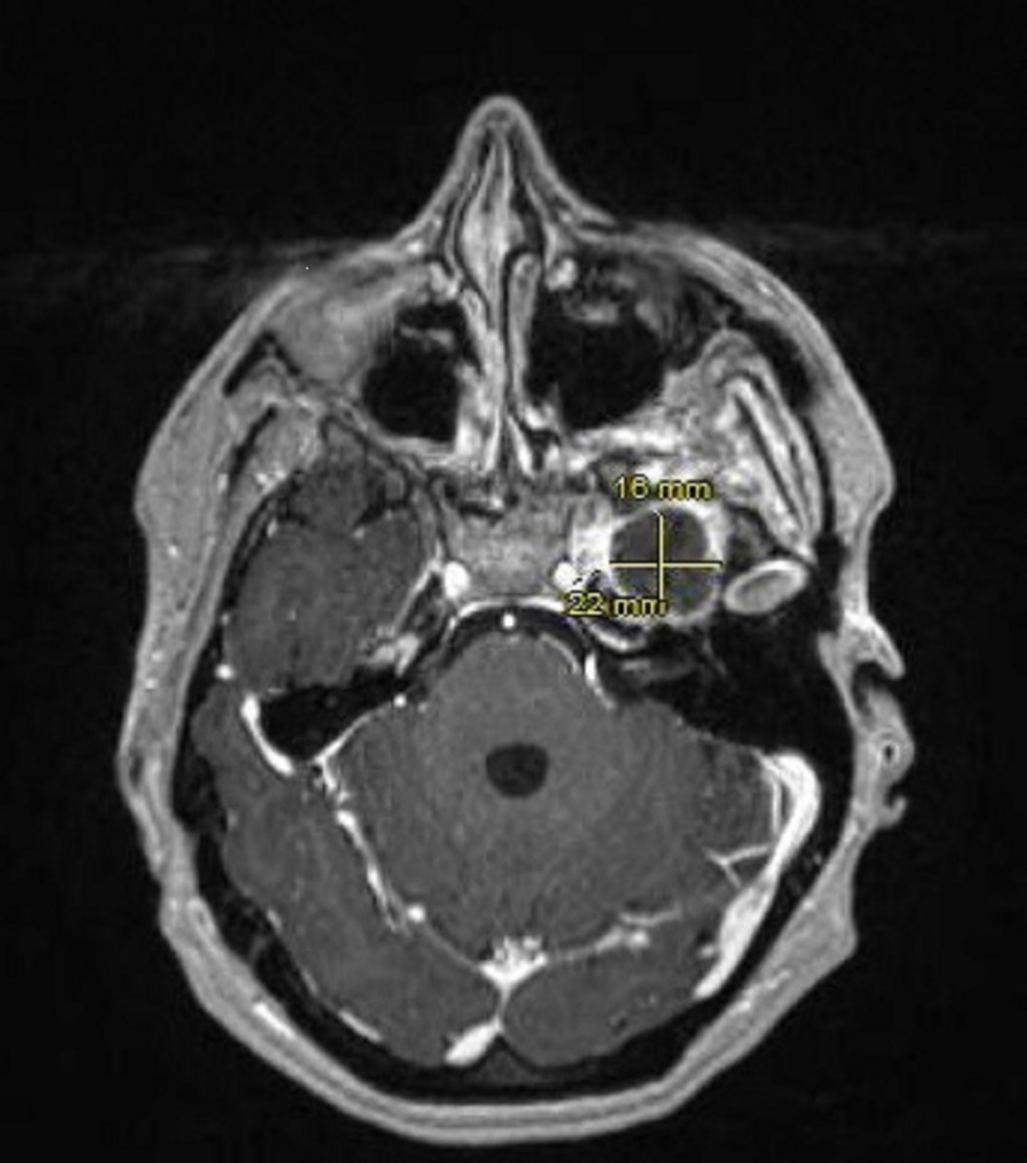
Magnetic resonance imaging (MRI) brain showing 22 x 16 mm soft tissue mass involving left trigeminal ganglion.
